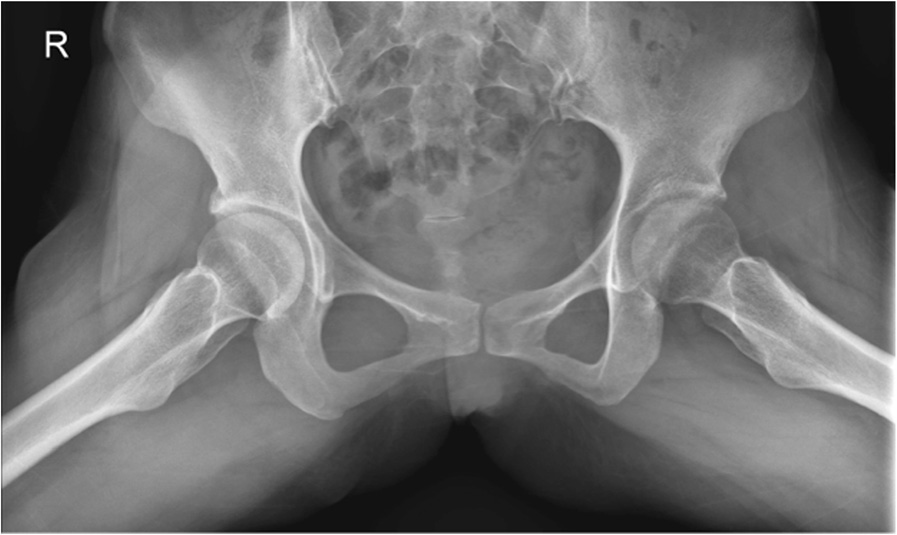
X-ray of her pelvis three months after surgery - Frog view showed mild osteopenic change over the left femoral head but joint space was preserved.
